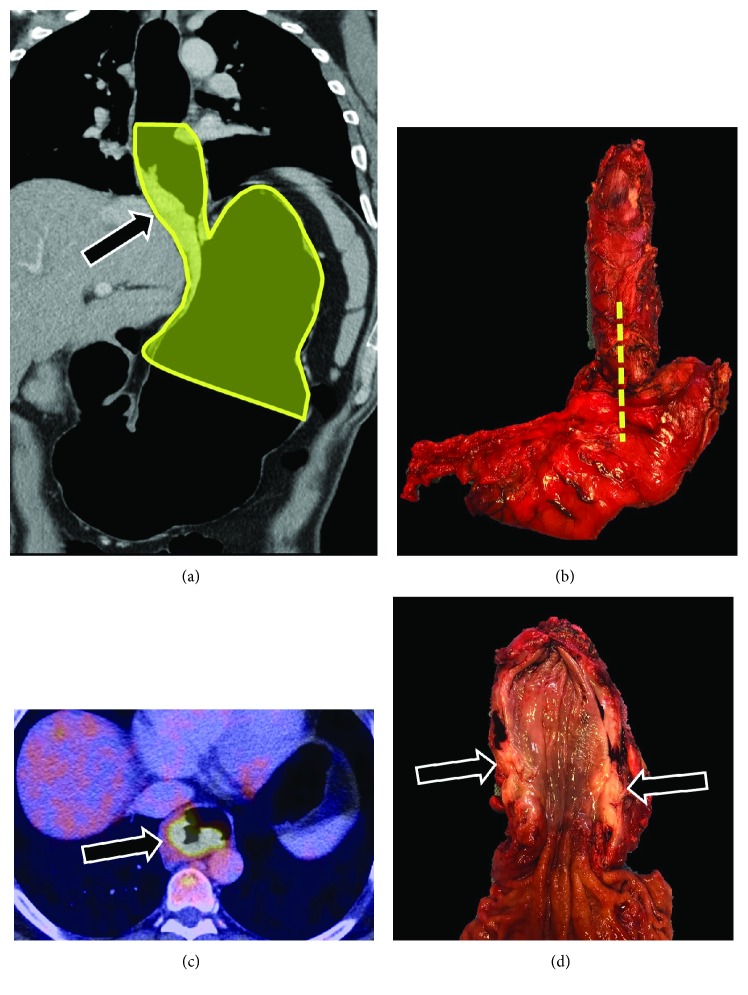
A 68-year-old male patient with EGJ confirmed adenocarcinoma (black arrows) showing a coronal curved reconstruction (a) with the planned surgery (yellow highlight), the axial pneumo-PET-CT fusion showing hypermetabolism of the tumor, and the surgical piece (b, closed, and d, opened).
